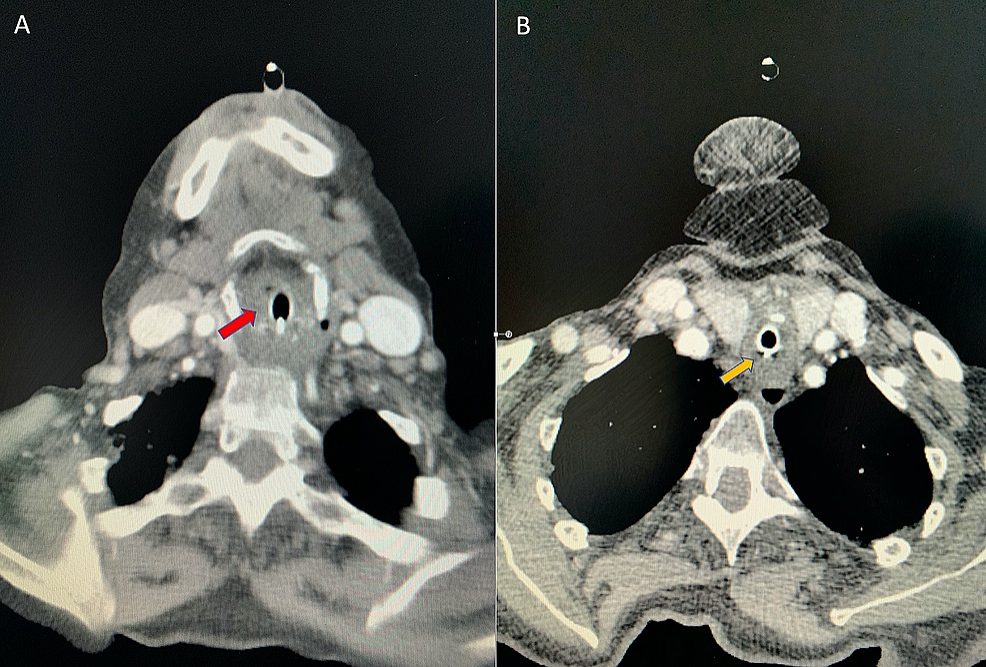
CT of the neck and thorax with contrast. (A) shows laryngeal oedema (red arrow) and (B) shows tracheal oedema (yellow arrow) resulting in severe airway narrowing. The trachea splinted open with an endotracheal tube. CT: computed tomography
